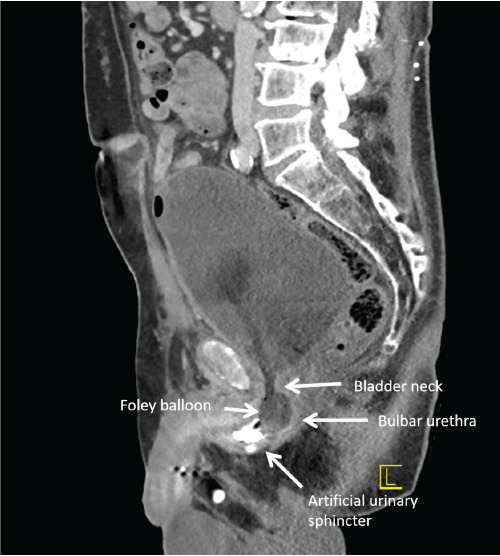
A sagittal computed tomography abdomen pelvis with intravenous contrast demonstrates a suprapubic catheter with inflated Foley balloon at the level of the bulbar urethra, just proximal to the artificial urinary sphincter. The bladder is also distended due to bladder outlet obstruction due to malpositioned catheter.
